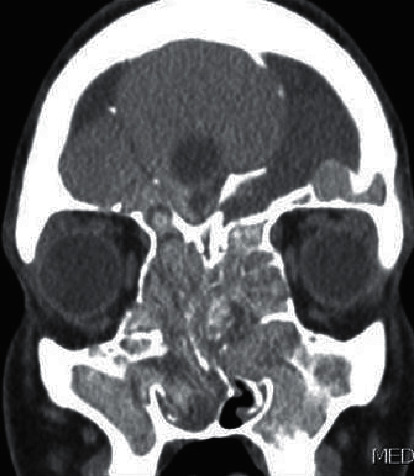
Computed tomography of the paranasal sinuses in the coronal plane, showing extensive disease with marked expansion of the sinuses, more pronounced in the right frontal sinus.
